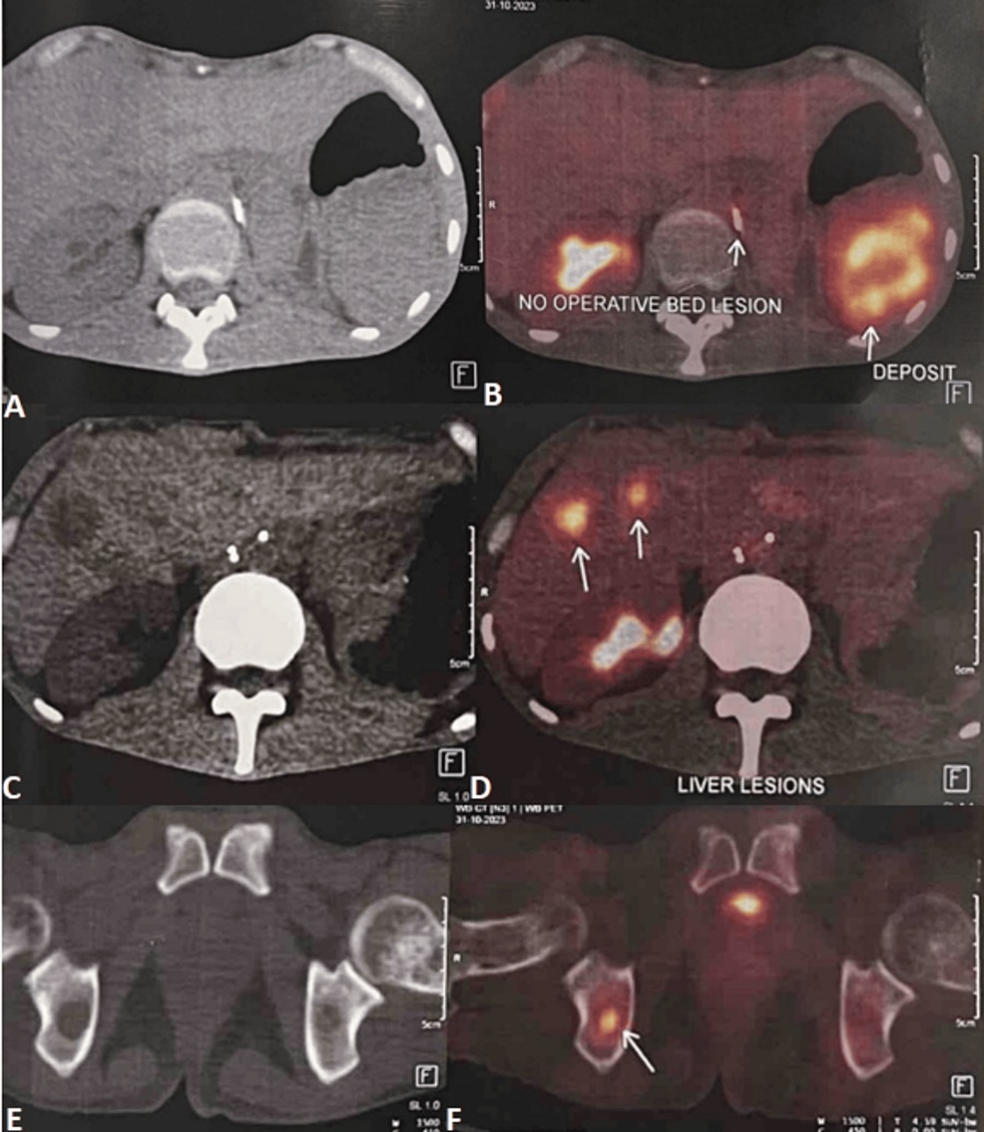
An 18F-FDG whole-body PET/CT scan reveals no lesions in the operative bed but shows FDG-avid hypodense perisplenic deposits (A, B, arrow), FDG-avid hypodense metastatic deposits in the liver (C, D, arrow), and an FDG-avid lytic lesion in the right ischium (E, F, arrow). F18-FDG: fluorine-18 fluorodeoxyglucose; PET: positron emission tomography
